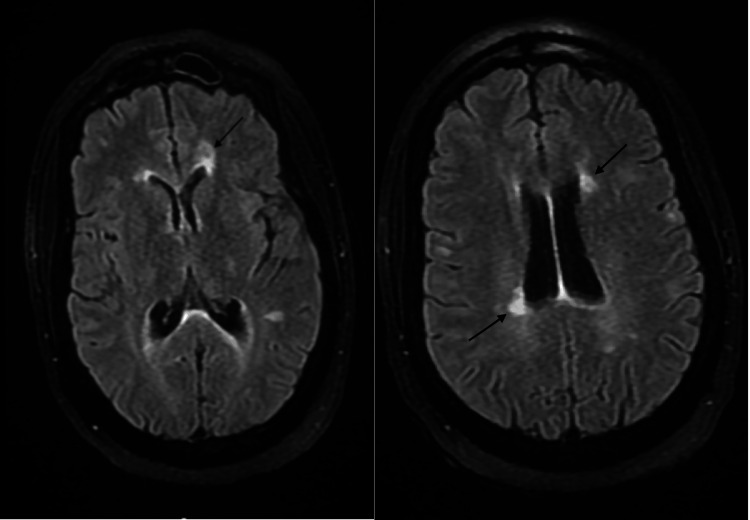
MRI brain T2 FLAIR showing multiple periventricular demyelinating lesions (black arrows) FLAIR: fluid-attenuated inversion recovery
